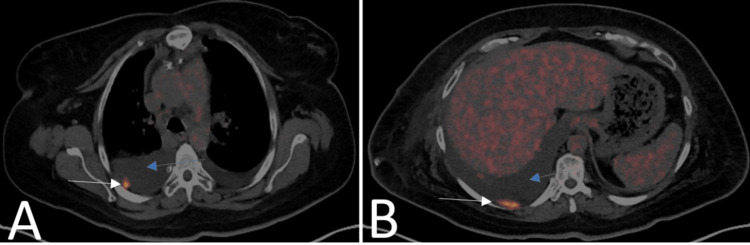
PET-CT scan of the chest The image shows multiple foci of FDG uptake in the pleura (white arrows) and pleural effusion (blue arrows) FDG: fludeoxyglucose; PET-CT: positron emission tomography-computed tomography
